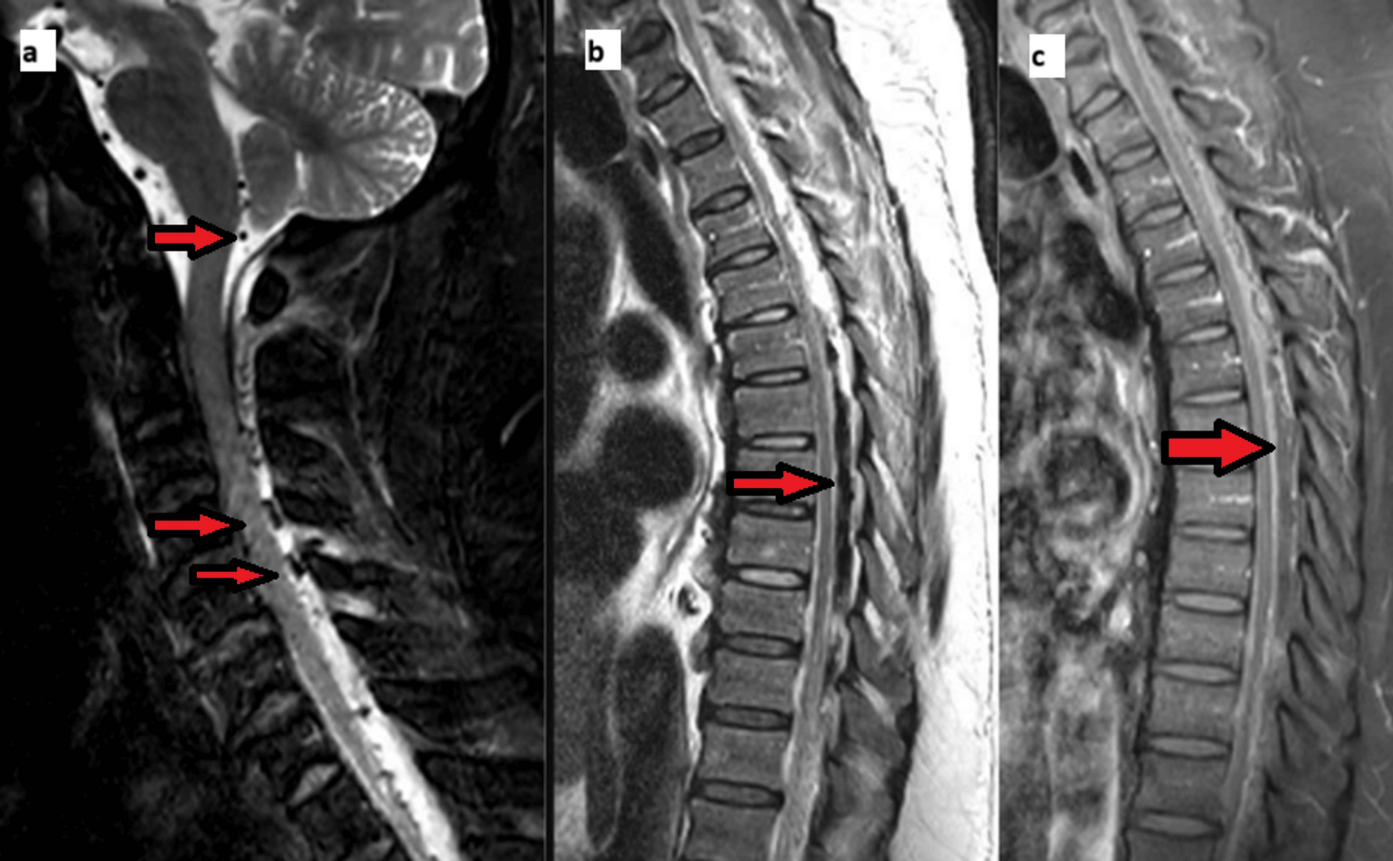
Magnetic resonance sagittal images showing long segment epidural fluid collection extending from the level of the foramen magnum to the visualized upper cervical spine, edema within the posterior neck soft tissues, left greater than right, long segment epidural fluid collection which extends the entire level of the spinal cord from the level of foramen magnum through the sacral canal. Heterogeneous epidural soft tissue signal intensity and enhancement is seen, with more localized air collection in the mid thoracic spine, diffuse enhancement of the epidural space and spinal dura, compatible with inflammatory change. (A) Dark signal on T1-weighted magnetic resonance imaging denotes foci of air (B) Long segment epidural fluid collection which extends the entire level of the spinal cord from the level of foramen magnum through the sacral canal. Heterogeneous epidural soft tissue signal intensity and enhancement is seen, with more localized air collection in the mid thoracic spine * (C) Long segment mass effect and compression of the CSF spaces within the thecal sac with anterior displacement of the spinal cord. Is greatest in the mid thoracic spine, with suspected focal signal changes within the cord at the level of T6.
